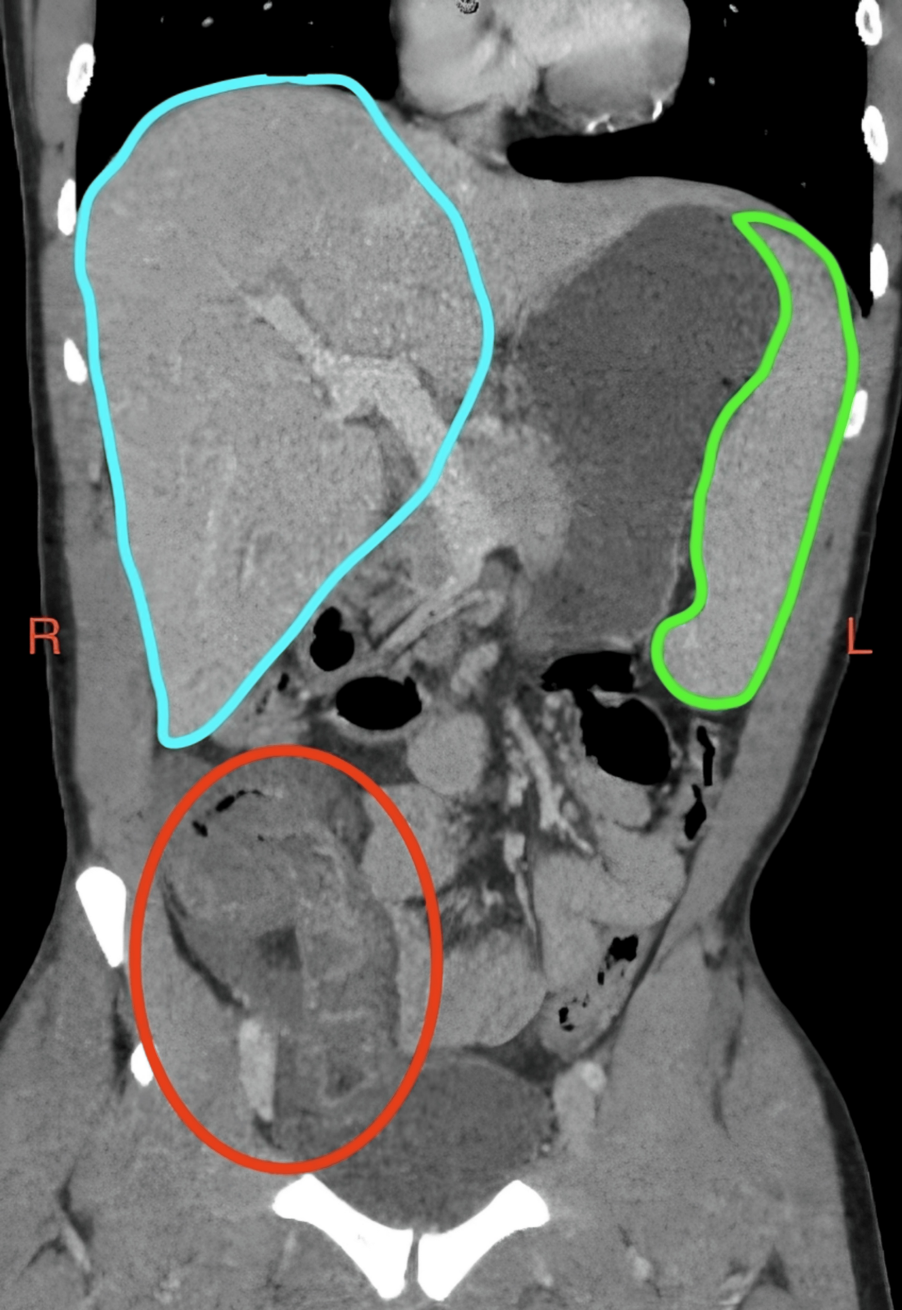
Coronal contrast-enhanced computed tomography of the abdomen and pelvis demonstrating hepatosplenomegaly and ileocolitis. Liver (blue) enlarged to 24 cm in craniocaudal dimension with no intrahepatic or extrahepatic biliary dilation. Spleen (green) enlarged to 17.3 cm in craniocaudal dimension. There is marked thickening of the wall of the terminal ileum, ascending colon, and cecum (red).
